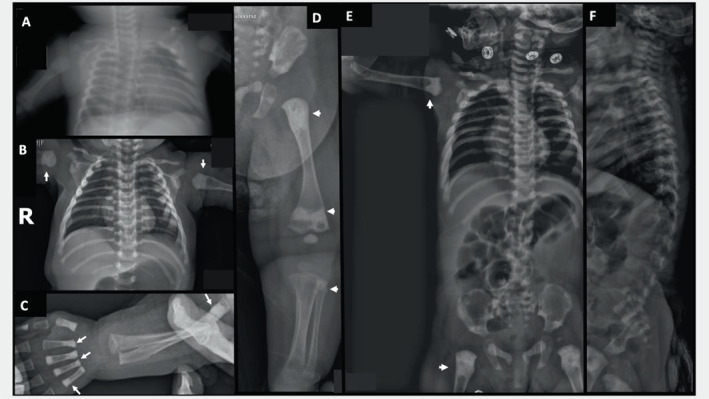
Patient 2. Radiographic findings during infancy. (*A*) At age 19 days, anteroposterior radiograph of the chest shows minimal osteosclerosis. (*B*) At age 2 months, marked metaphyseal osteosclerosis affects the proximal humeri (arrows). The clavicles, ribs, scapulae, and spine are osteosclerotic. (*C*) At age 2 months, metaphyseal osteosclerosis had faded in the distal left radius and ulna, possibly due to healing fractures there, but is apparent in four metacarpals (arrows) and proximal ulna (arrow). (*D*) At age 2 months, the dense metaphyseal osteosclerosis is no longer prominent at the provisional zone of calcification in the distal femur, and dense metaphyseal osteosclerosis is absent at the ends of the tibia (arrows). The proximal tibia and fibula (arrow) show healing fractures. Osteosclerosis is peripheral in the iliac bone and focal in the ischium and pubis. (*E*,*F*) At age 6 months, anteroposterior and lateral views of the spine demonstrate thick and osteosclerotic ribs and osteosclerosis of the clavicles, scapulae, proximal humeri, spine (especially the cervical region), and pelvis.
